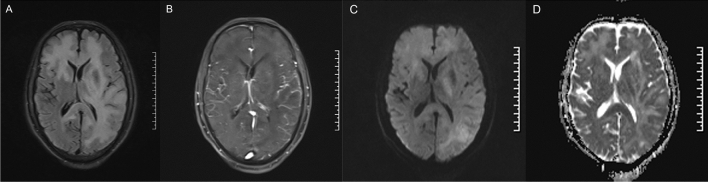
Brain MRI at initial diagnosis in a 75 year-old male patient. T2FLAIR, A showed hyperintensity in the right frontal lobe and the left frontal, parietal and temporal lobe, and T1 enhancement, B sequences showed patchy mild enhancement. DWI (C) and ADC (D) sequences showed hyperintensity lesions
